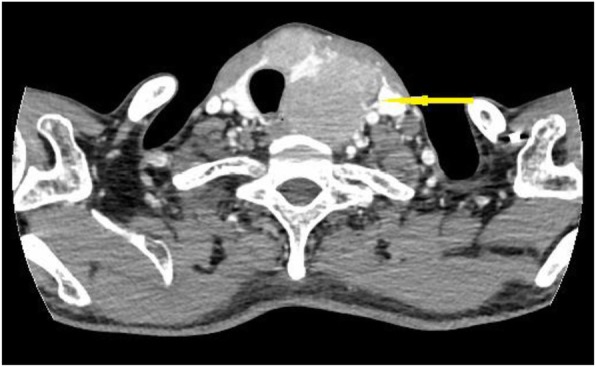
CT scan showed large masses in the isthmus and left lobes of the thyroid
